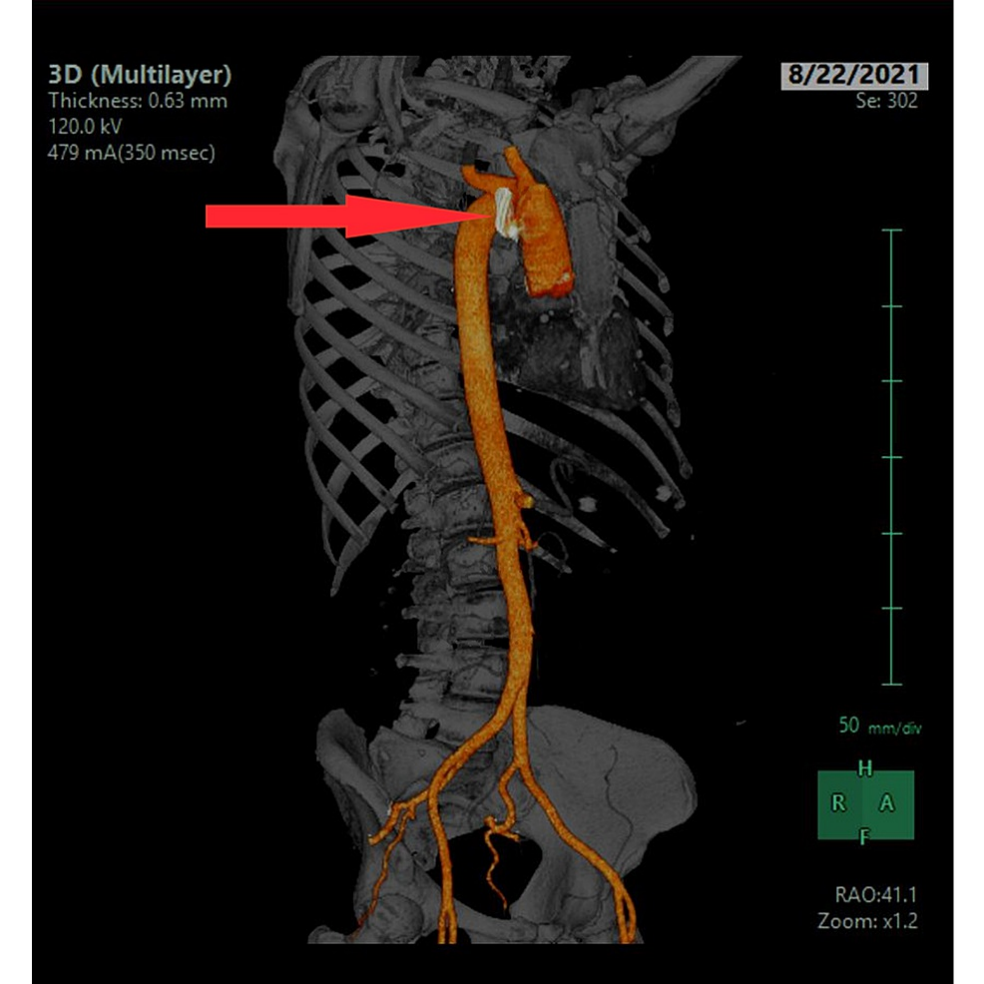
Computed tomography angiogram of the chest, abdomen, and pelvis The red arrow is pointing towards the lesion.
